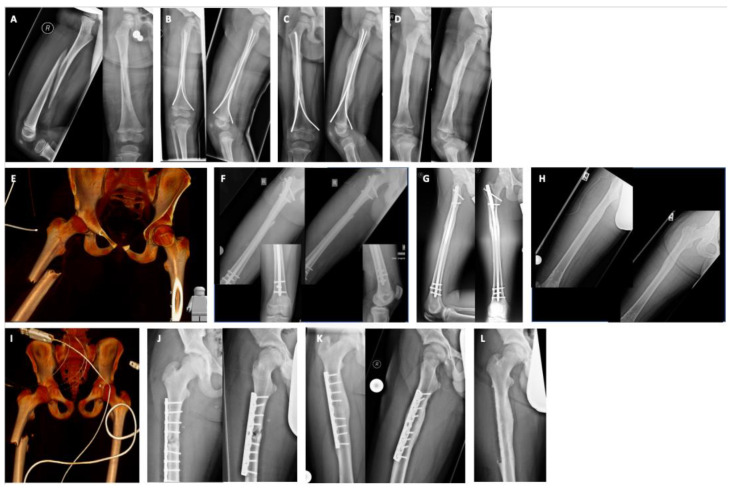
Different treatment strategies in children with femur fracturea. X-ray series demonstrating fracture type, treatment and bone healing process. (A–D): One-year-old boy twisted the leg while playing: 32-D/5.1 (A), ESIN (B) with secondary bone healing (C) and after hardware removal (D). (E–H): Sixteen-year-old boy after traffic accident with collision (moped against car): 32-d/4.1 (E), IMN (F) with mainly primary bone healing (G) and after hardware removal (H). (I–L): Twelve-year-old boy after traffic accident with collision (bicycle against car): 32-D/4.1 (I), ORIF (J) with mainly primary bone healing (K) and after hardware removal (L).
